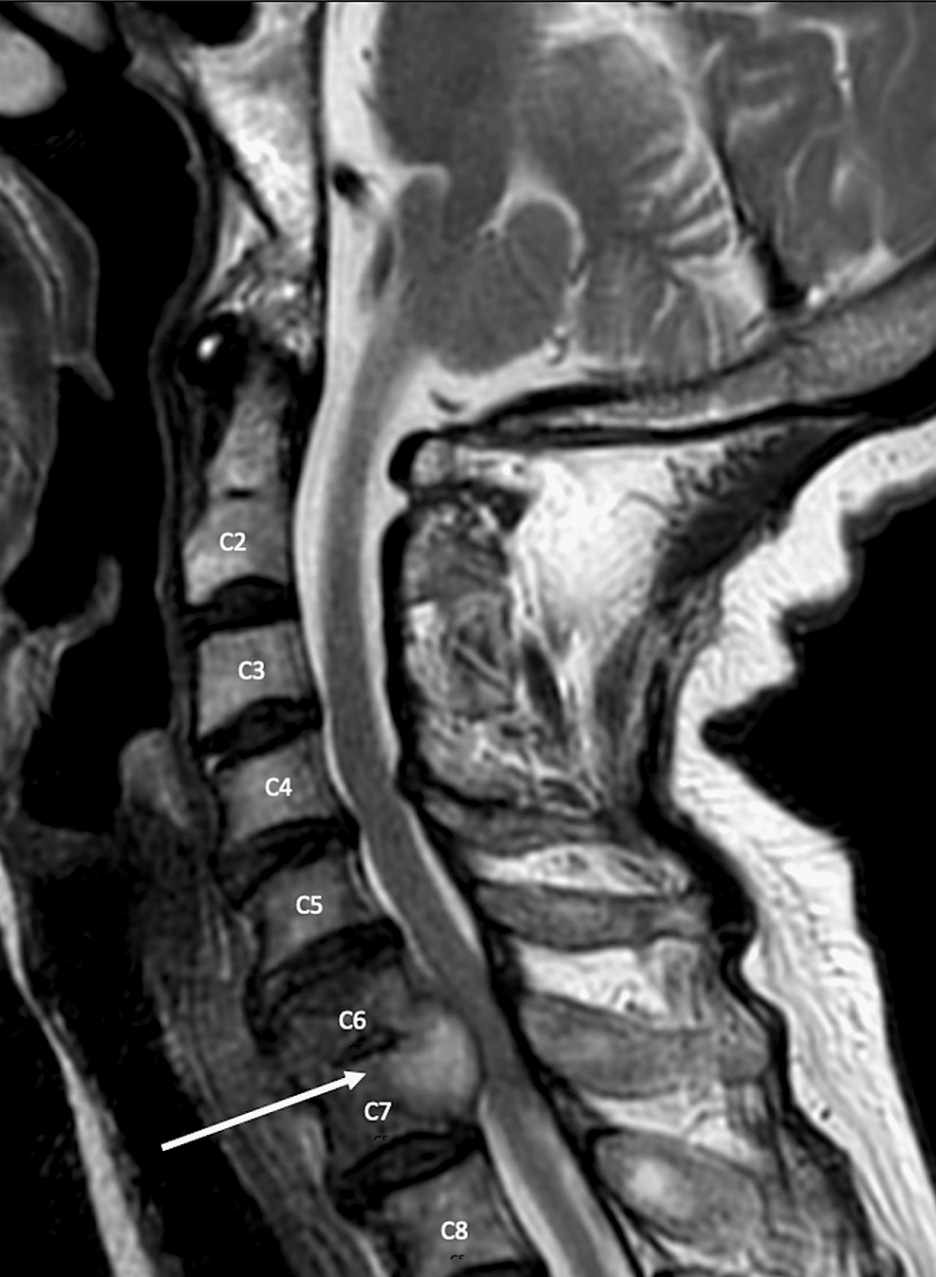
Sagittal cervical spine MRI portraying compressive collapse of the C6/C7 vertebrae, stenosis, and cord compression
